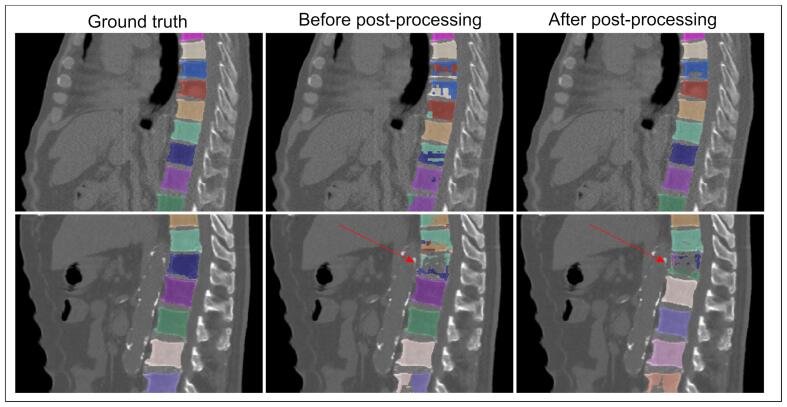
Examples of automatic delineations (using the combined approach) before and after post-processing, projected on the corresponding sagittal CT image in bone setting (W = 2500, L = 1000). Different colors represent different labels. Top row: mixed and incorrect labels in a part of the spine are corrected by post-processing. Bottom row: due to incorrect segmentation of one vertebra (arrows), all vertebrae below are labeled incorrectly even though they were largely correct before.
